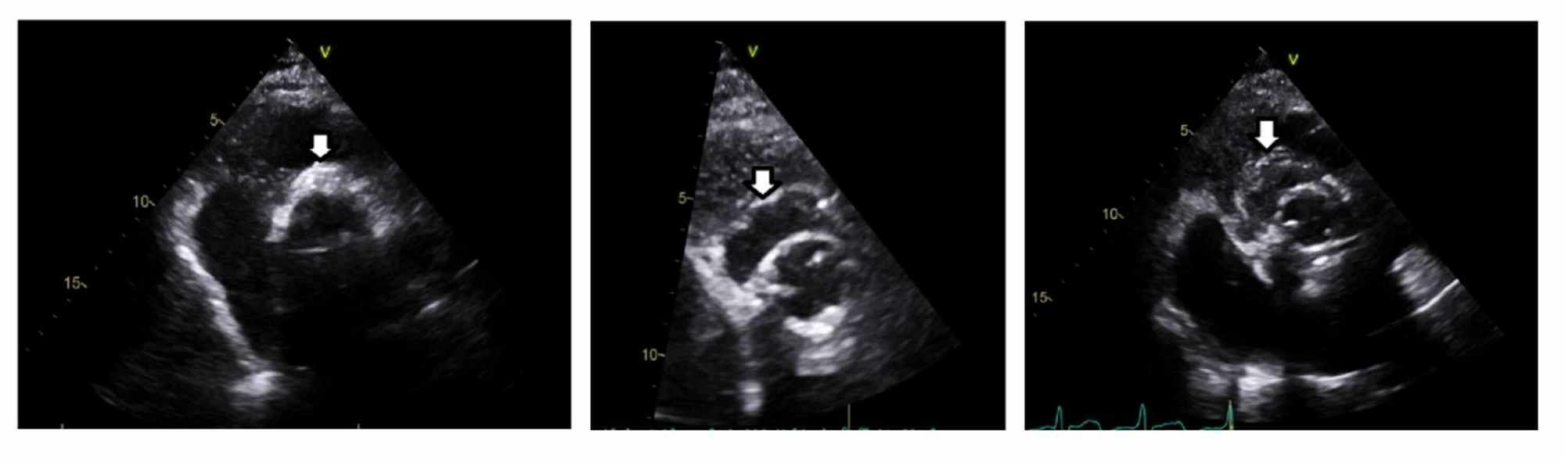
Transthoracic echocardiogram, parasternal, short axis view, aortic valve Leftmost image: increased echogenicity of the annulus of the bioprosthetic aortic valve, but no definite abscess (arrow). Middle image: development of paravalvular abscess. Rightmost image: echolucent area, suggestive of an increase in the abscess size.
